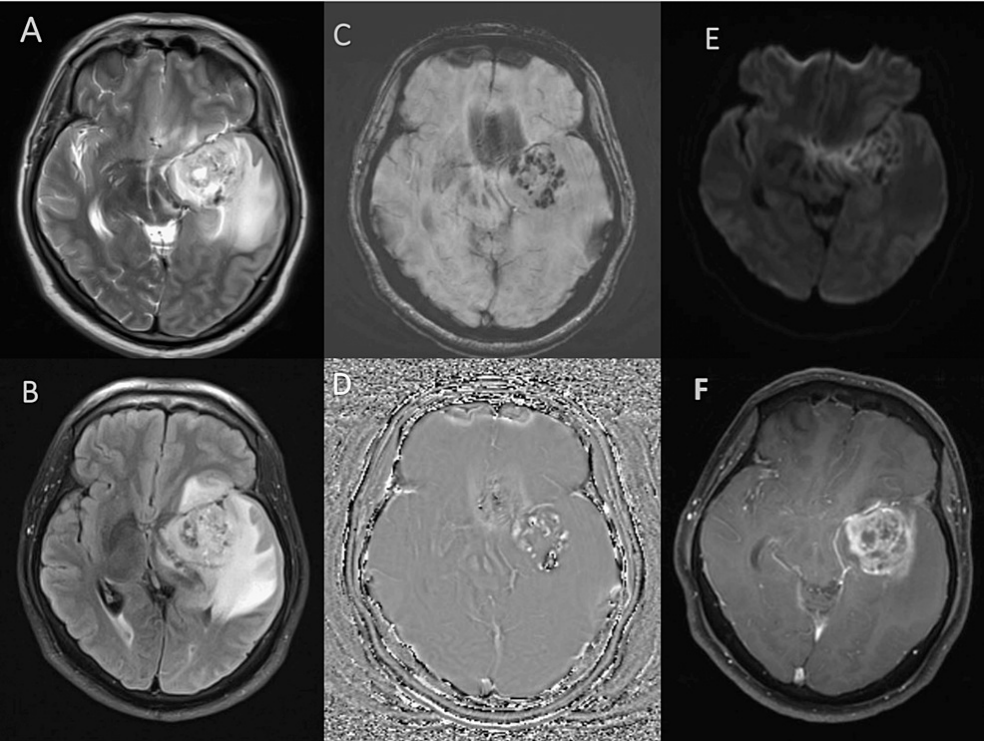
MRI image of left temporal glioblastoma with significant mass effect, areas of diffusion restriction, punctate areas of hemorrhage, moderate perilesional edema, and thick peripheral enhancement. (A: T2WI, B: FLAIR, C: SWI magnitude image, D: Filtered phase image, E: DWI, F: T1FS post-contrast image.) DWI: Diffusion-weighted imaging, FLAIR: Fluid attenuated inversion recovery, SWI: Susceptibility weighted imaging, T1FS: T1 weighted fat-saturated image, T2WI: T2 weighted image
